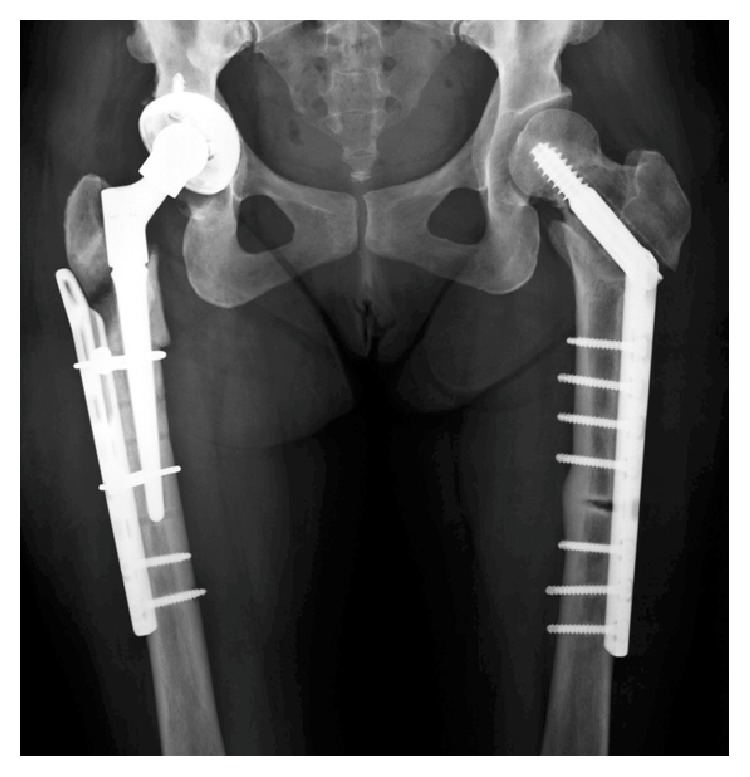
Radiograph of right hip one year after surgery, showing no migration of the implant.
